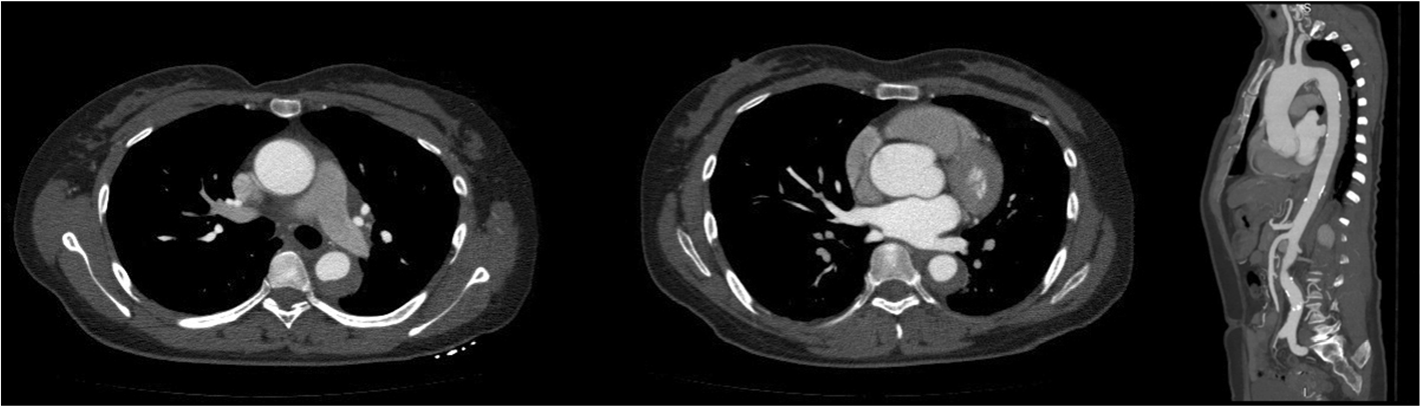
Initial chest CTA showing intramural hematoma around proximal part of the descending thoracic aorta
